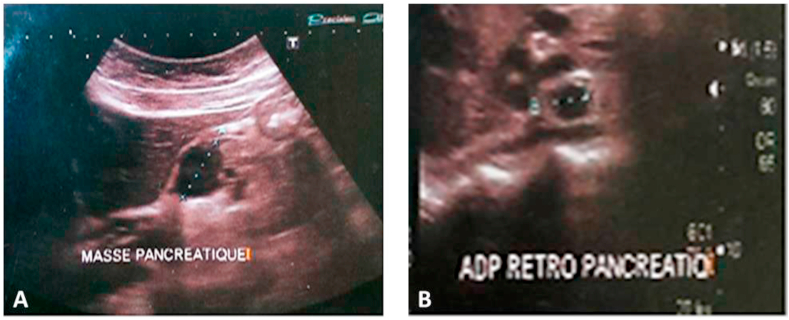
Abdominal ultrasound showing a 40-mm mass in the pancreatic body (A) with multiple peripancreatic lymphadenopathy (B).
